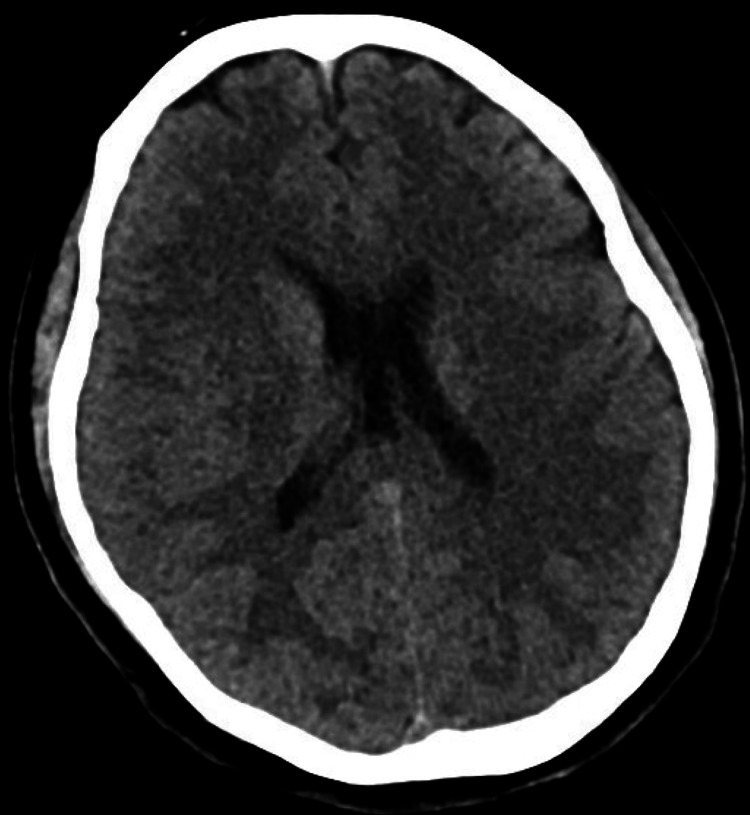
A non-contrast computed tomography (CT) of the head of a patient with poppers-induced methemoglobinemia showed no acute intracranial abnormality
